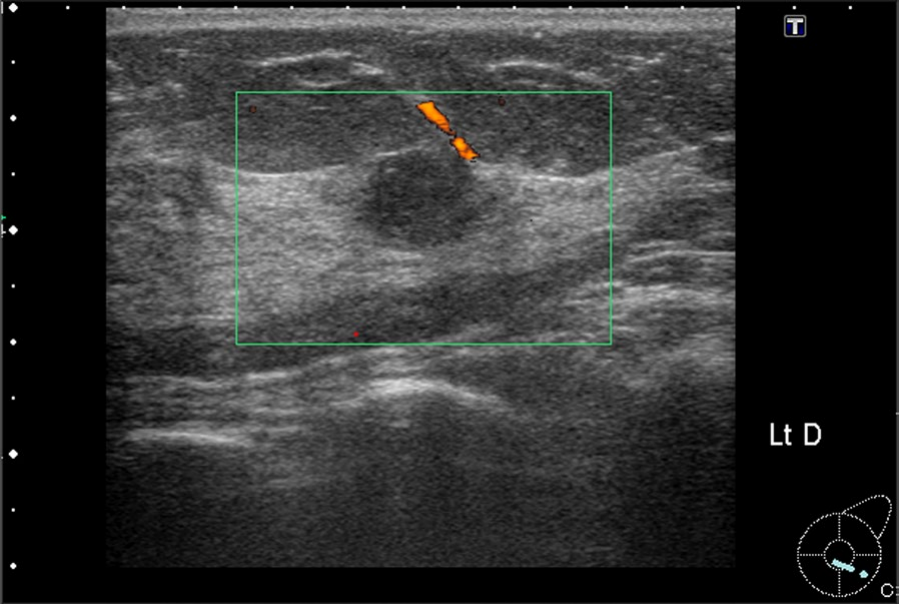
Ultrasonography. A 1.2-cm-diameter relatively smoothly marginated hypoechoic mass with poor internal blood flow
